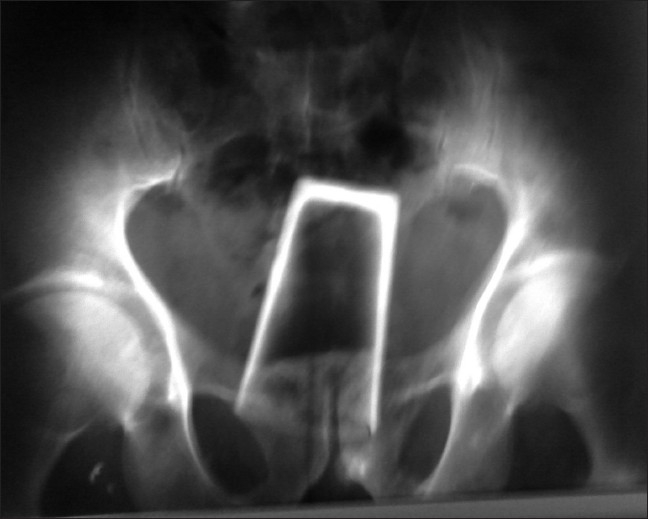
X-ray pelvis showing the glass in lower abdomen and pelvis
